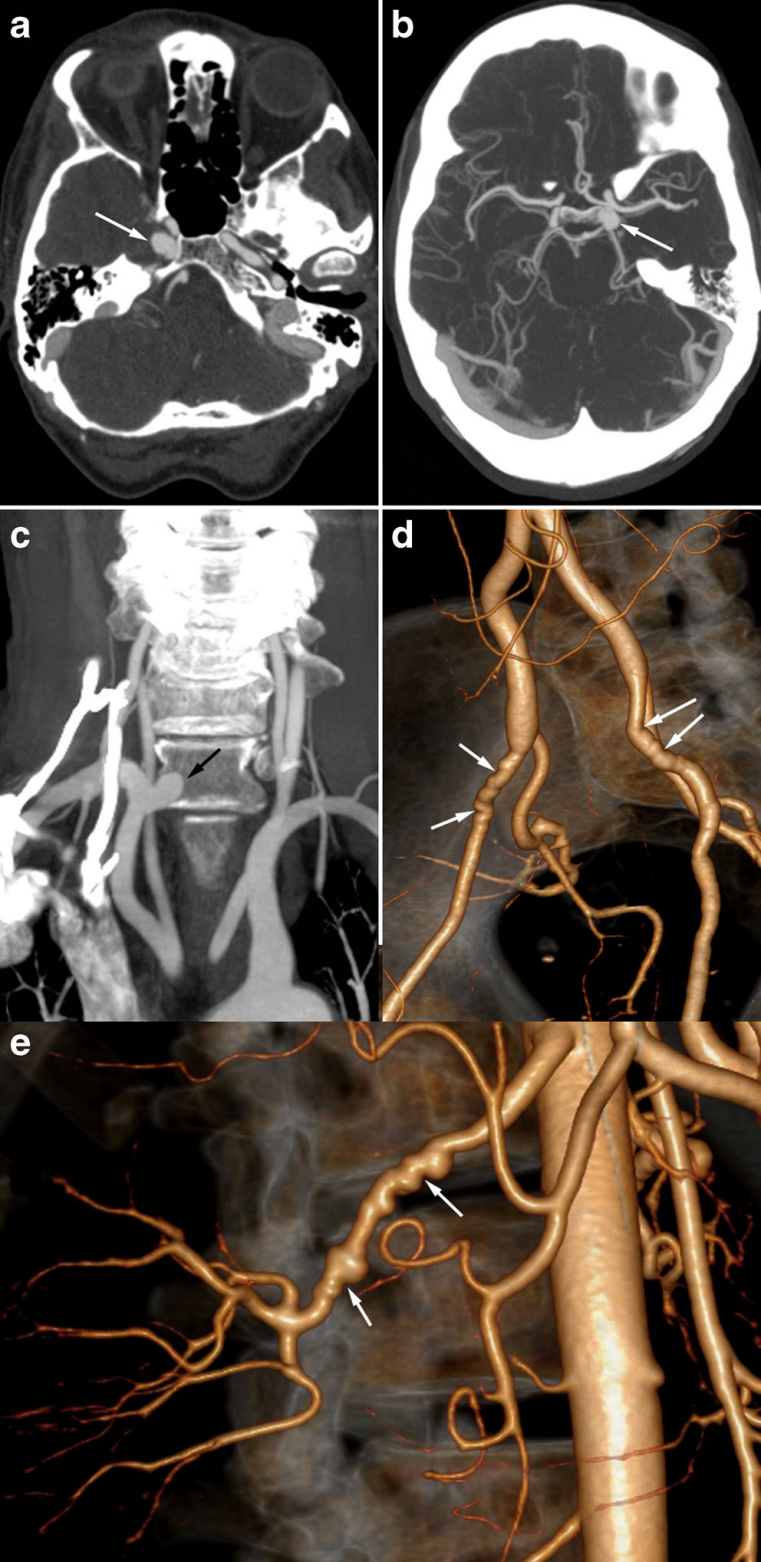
Radiologic findings in a 56-year-old female patient presenting with non-specific headaches. a and b Cerebral CTA showing bilateral carotid aneurysms with intracavernous development (*white arrows*). c Coronal CTA MIP reconstruction of the supra-aortic trunks, showing a right subclavicular aneurysm (*black arrow*). d and e Three-dimensional volume-rendered abdominal CTA showing typical medial FMD affecting the external iliac arteries (*white arrows*, d) and the right renal artery (*white arrows*, e)
